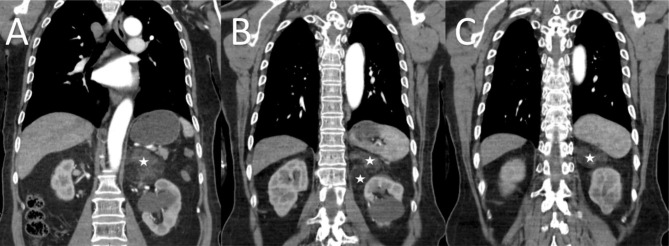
Coronal section of preoperative computed tomography (CT) scan; (A) anterior mass, (B) both masses, (C) posterior mass
